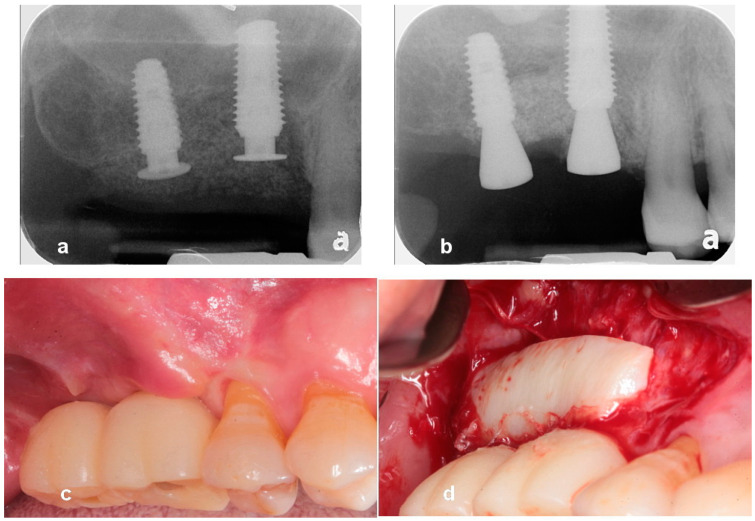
(a) A preoperative periapical radiograph. (b) A plain radiograph after uncovering procedure reveals an augmented ridge over implant platform. (c) Note the insufficiently attached keratinized gingiva around the implant restoration. (d) Suture-less free gingival graft was performed to widen the zone of the attached gingiva.
